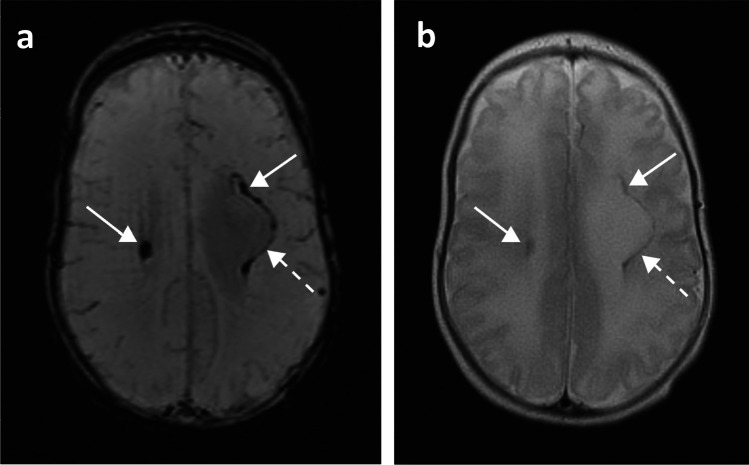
A 14-week-old boy born extremely premature at 26 gestational weeks with magnetic resonance imaging performed at term-equivalent age. a Axial susceptibility-weighted image shows bilateral germinal matrix haemorrhages (*arrows*) with susceptibility-related signal loss, and sequelae of a broad-based porencephalic cyst on the left side (*broken arrow*) with adjacent susceptibility-related signal loss. b Axial T2-weighted image shows bilateral germinal matrix haemorrhages as hypointensities (*arrows*) and sequelae of a broad-based porencephalic cyst on the left side (*broken arrow*) with adjacent hypointensities
